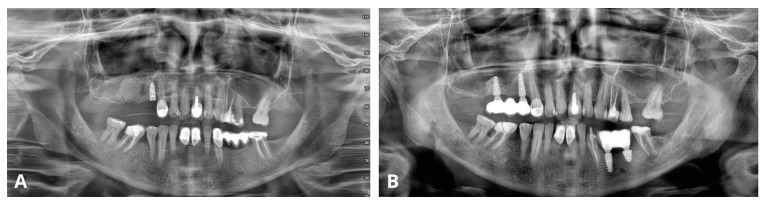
Comparison of orthopantomogram (OPG) immediately after the procedure (A) and 4 years after delivering the final reconstruction on implants (B).
